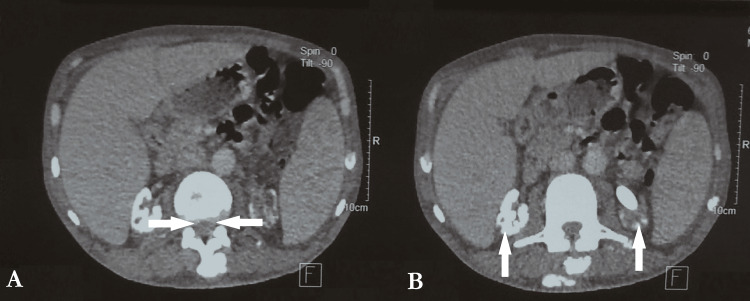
Abdominopelvic CT scans revealing lumbar vertebral compression and nephrocalcinosis. (A) Lumbar vertebral compression. (B) Nephrocalcinosis.
